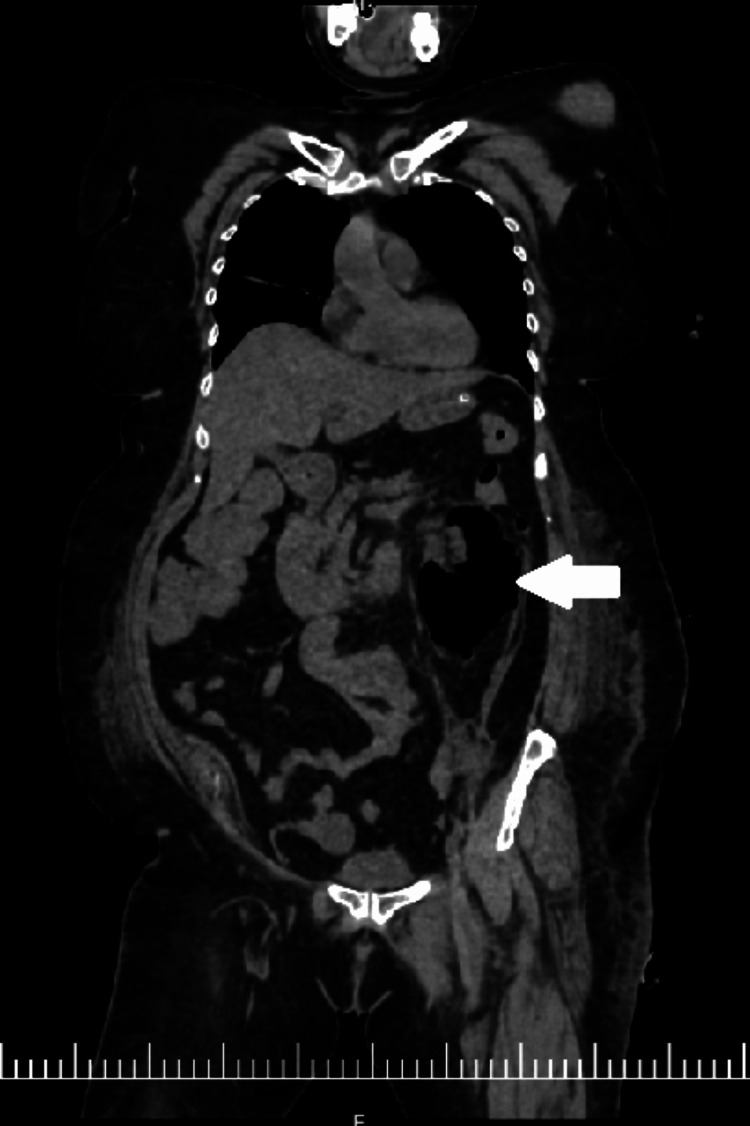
Computed tomography (CT) images of the kidneys, ureters, and bladder demonstrating a bulky left kidney with ill-defined collections and perinephric fat stranding, consistent with left emphysematous pyelonephritis.
